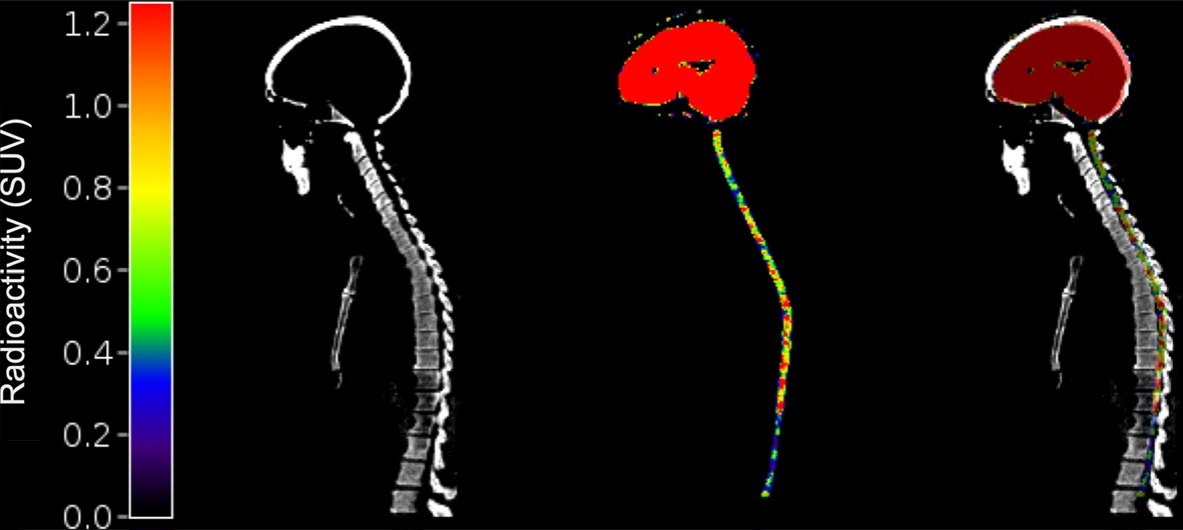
Sagittal view of a CT image, a PET image (SUV, 30–60 min), and overlaid CT/PET of the Full Central Nervous System (brain + SC)
